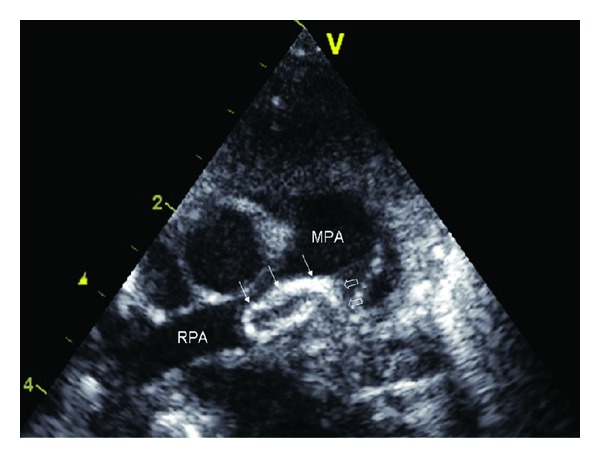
Cross-sectional echocardiogram showing the protrusion of the echodense mass (arrows) into the right pulmonary artery, extending from obstructed left pulmonary artery (block arrows). MPA: main pulmonary artery. RPA: right pulmonary artery.
